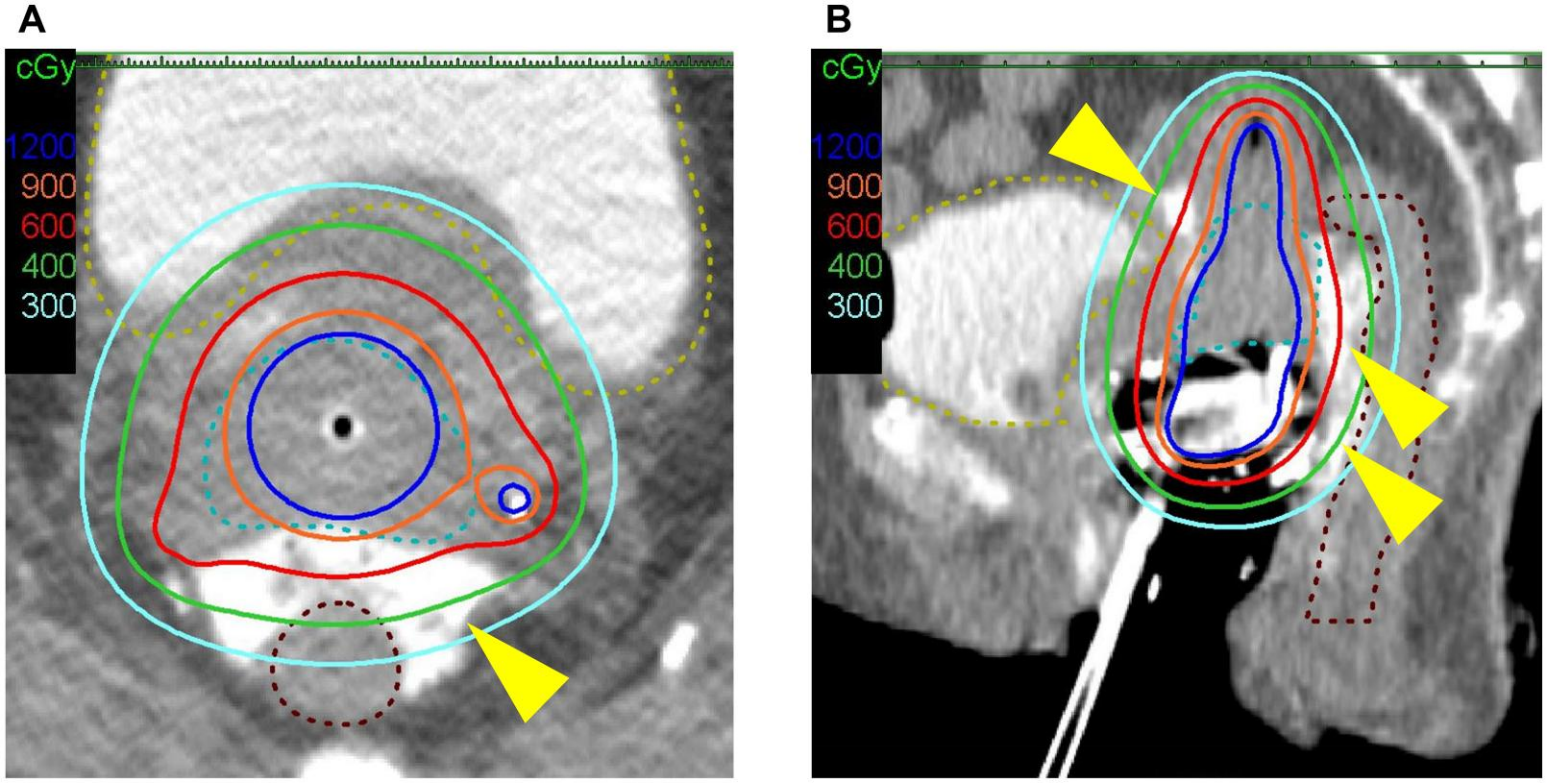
Isodose curves for cervical uterine brachytherapy using a gel spacer. (A) Axial view. (B) Sagittal view. Yellow dashed line: bladder. Brown dashed line: rectum. Light blue dashed line: high-risk clinical target volume. A gel spacer (MucoUp^®^; arrowhead) was inserted between the anterior vaginal wall and the bladder (vesicovaginal septum) and between the posterior wall of the vagina and the rectum (rectovaginal septum) with transrectal ultrasound guidance. After spacer insertion, the distance between the CTV_HR_ and the rectum was 9-12 mm on treatment planning CT. The MucoUp prevents the 6-Gy isodose line from crossing the contours of the bladder and rectum.
